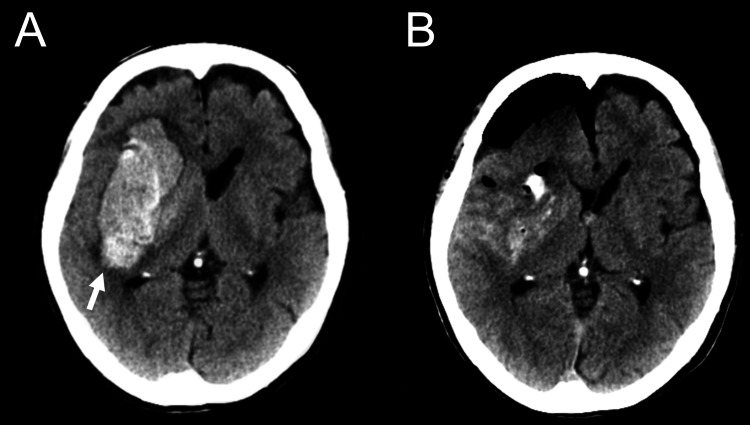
Preoperative and postoperative CT images of case 1. A 70-year-old female patient was admitted to our institution with left paralysis and loss of consciousness. CT imaging revealed an intracerebral hemorrhage of 89.0 ml in the right putamen (A). Subsequently, endoscopic hematoma evacuation was performed under local anesthesia. Postoperative CT imaging revealed that 95.8% of the hematoma had been successfully removed (B).
